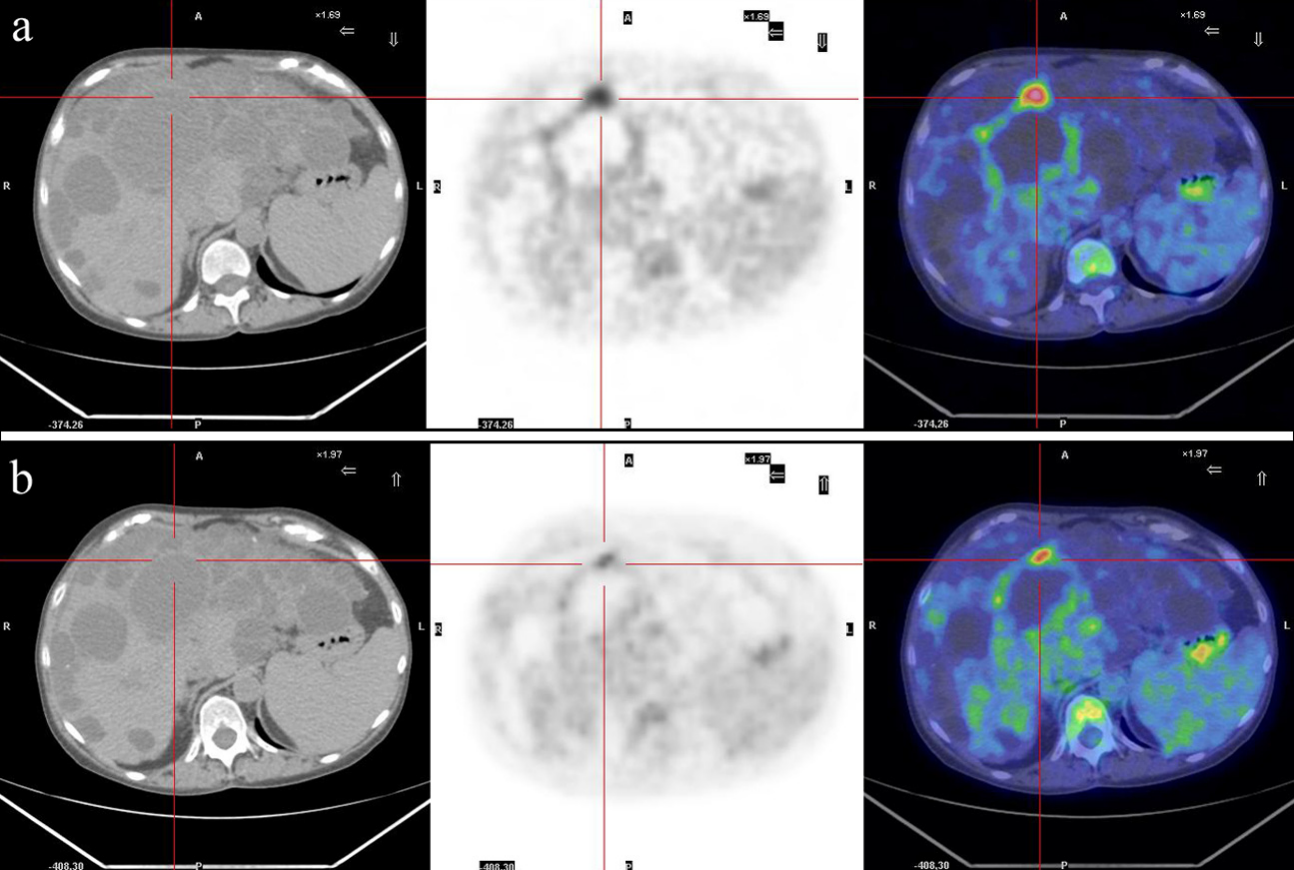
FDG-PET scan: intracystic infection in a large liver cyst in a ADPKD patient, before and after antibiotic therapy.
